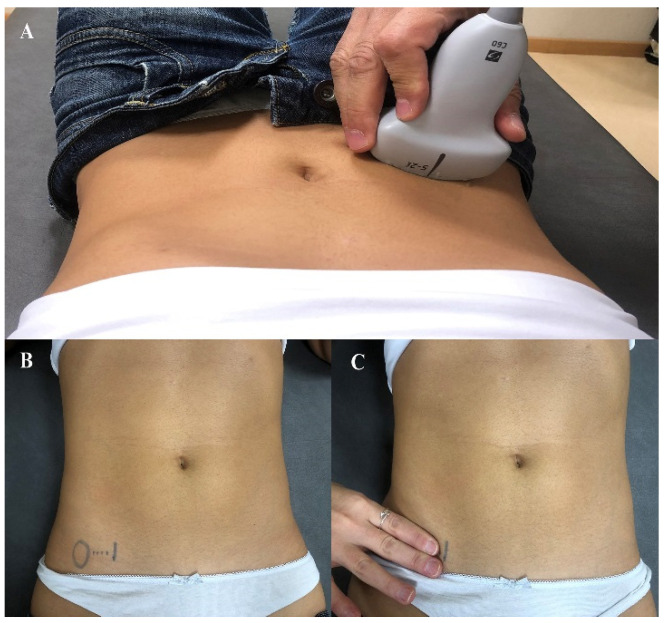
Ultrasound probe placed transversely just above the iliac crest in the midaxillary line (A); Manual palpation of the transversus abdominis by placing the index and middle fingers just 2 cm (B); medial to the anterior superior iliac spine (C).
